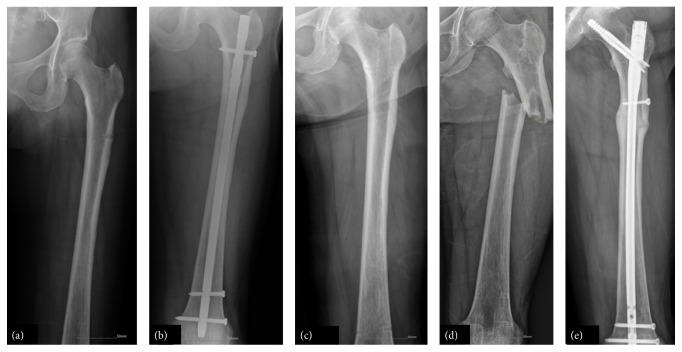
(a) Initial radiograph of a 64-year-old woman shows an atypical fracture of the subtrochanteric region of the femur. She had no history of antiosteoporotic medication. (b) Intramedullary nailing was performed. (c) After complete healing, implanted nail was removed 12 months after initial surgery. (d) Refracture occurred 33 months after nail removal. (e) A radiograph at 12 months after surgery shows complete healing.
